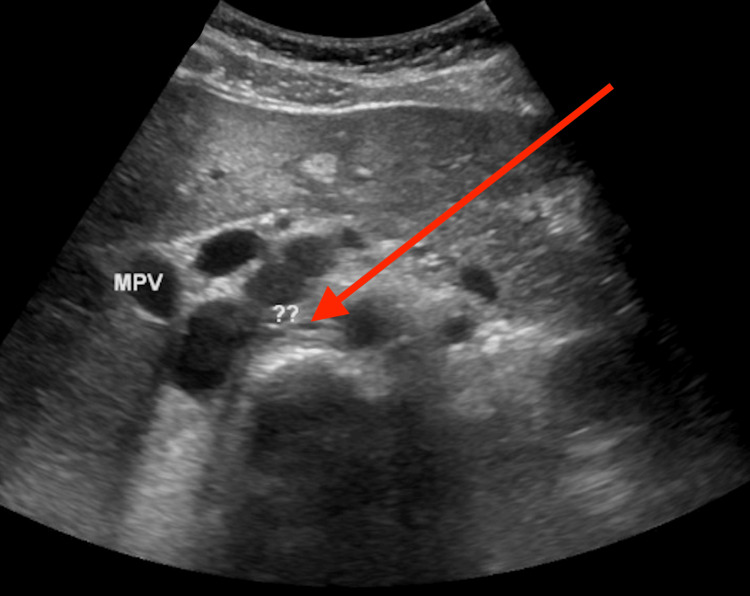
Suprarenal mass on ultrasonography The arrow refers to the suprarenal mass.
